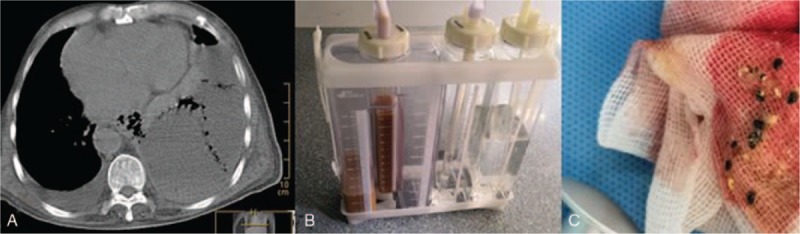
(A) HRCT image of the left pleural cavity showing pleural effusion with air in it. (B and C) Thoracic closed drainage confirmed food residue in it.
